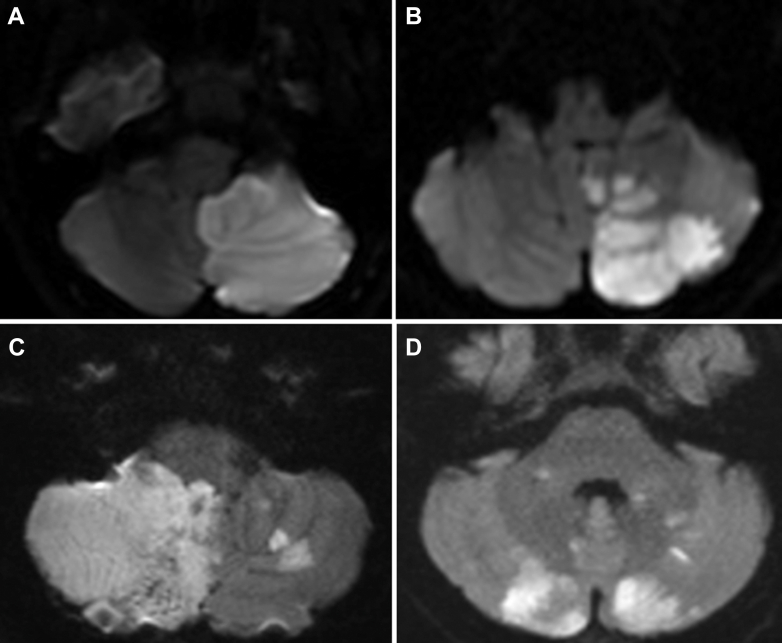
Brain MRI DWI (A) Brain MRI DWI of case 1 revealed a large left cerebellar ischemic stroke. (B) Brain MRI DWI of case 2 showed a large left cerebellar ischemic stroke. (C) Brain MRI DWI of case 3 showed a large right and small left cerebellar ischemic stroke. (D) Brain MRI DWI of case 4 showed multifocal ischemic stroke involving bilateral cerebellar hemispheres. DWI = diffuse weighted imaging; MRI = magnetic resonance imaging.
